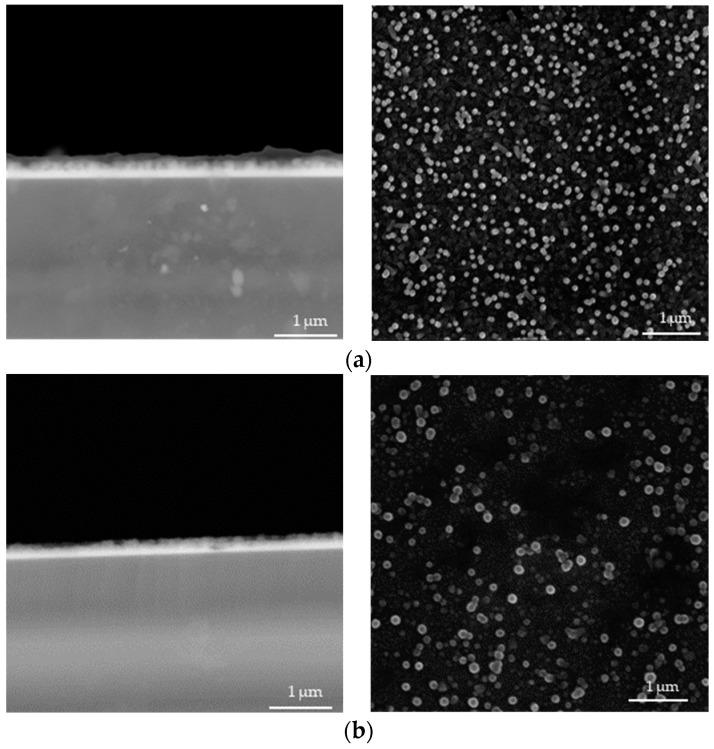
SEM images of nanorods obtained without ultrasounds and dried for 1.5 h (a) and 2.0 h (b) (left: cross-section image; right: surface image).
